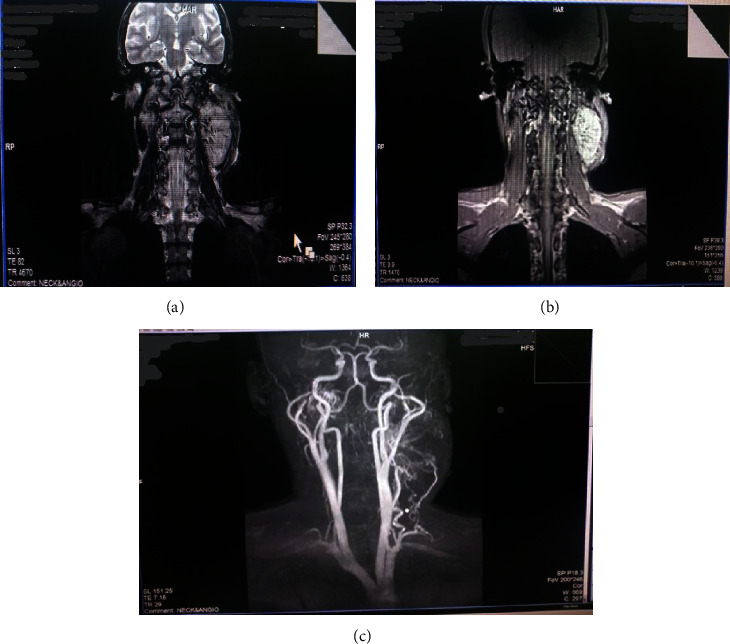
(a, b) An MRI image of the same mass. (c) MRI angiography demonstrates a highly vascular mass splaying the internal and external carotid arteries.
